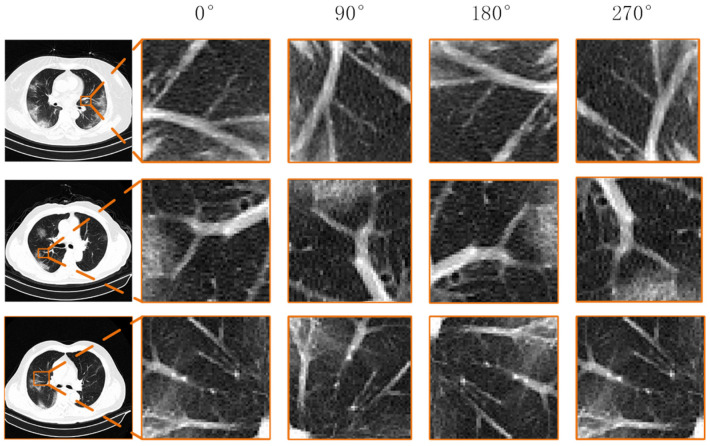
Example of blood vessel orientation and rotation in CT images of COVID-19 patients.
